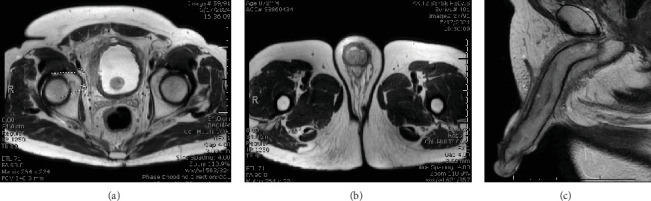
(a) Pelvic MRI highlighting the 1.6 cm right lateral bladder wall defect adjacent to the TURBT defect. (b) There was increased diffusion restriction of the R corpus cavernosum initially hypothesized to be secondary to infection or malignancy. (c) Sagittal view shows engorgement of the corporal bodies without obvious tumor invasion.
